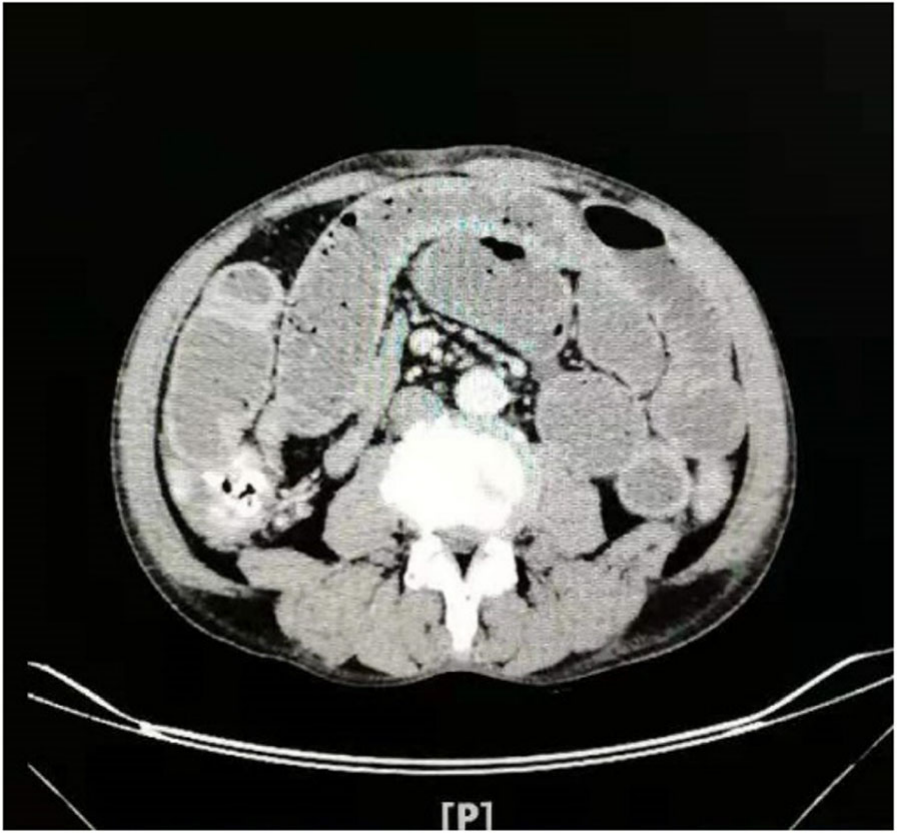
Preoperative abdominal CT examination showed small bowel obstruction
